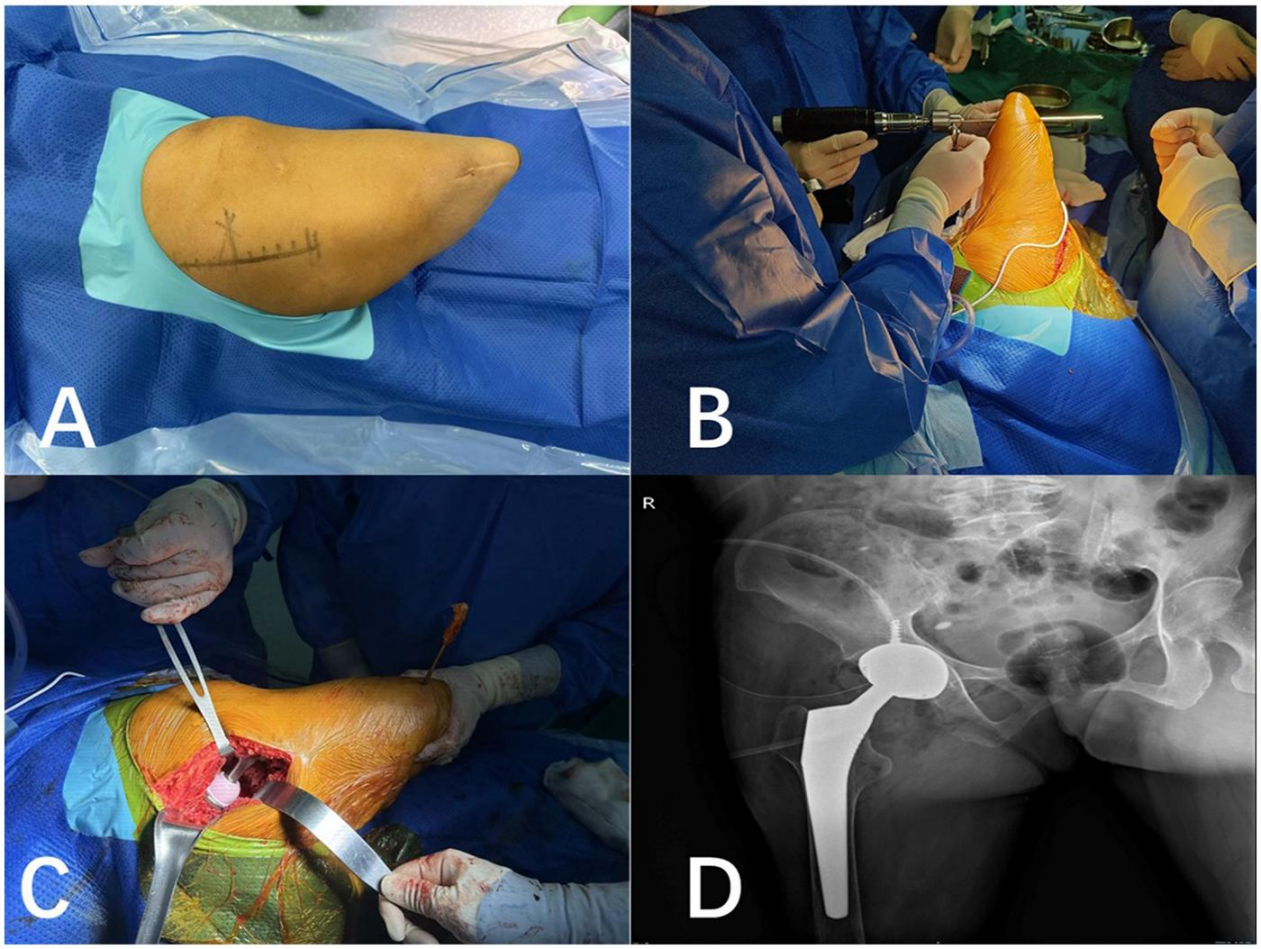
(A) the posterior lateral surgical incision of the right hip joint. (B)The Steinmann pin inserted in the distal femur. (C) Intraoperative image showing the THA for the patient. (D) Postoperative radiographs revealed satisfactory.
